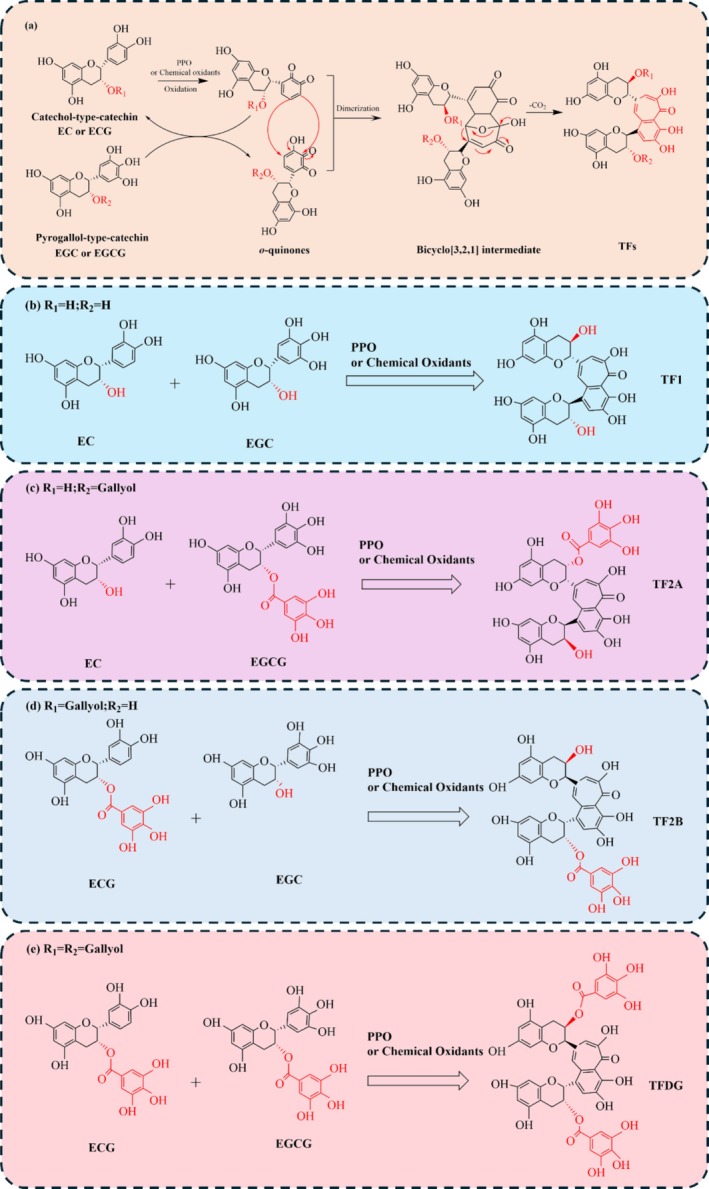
(a) The formation mechanism of PPO‐catalyzed theaflavin from catechins; pathways for the synthesis of four main theaflavins: (b) TF1; (c) TF2A; (d) TF2B; (e) TFDG.
